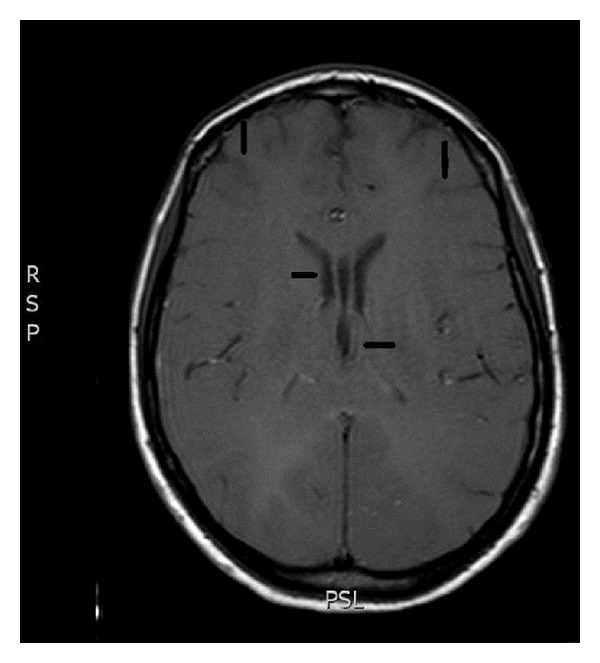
Brain MRI (T1-weighted image) showed diffuse swelling of the brain due to extensive edema of the white matter characterized *loss* of gray-white junction and collapse of the ventricles (arrows).
